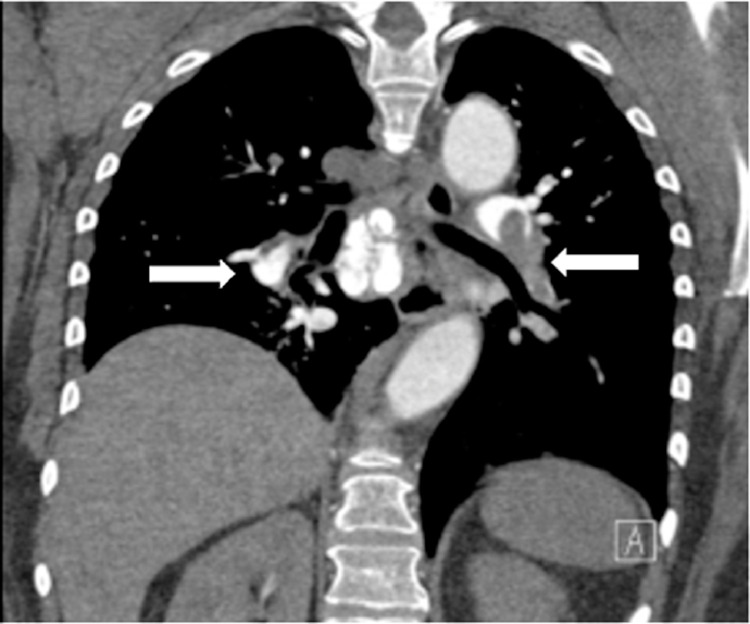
(Case 1). CT imaging demonstrated bilateral segmental and subsegmental pulmonary emboli within the bilateral upper, middle, and lower lobe (arrows).
